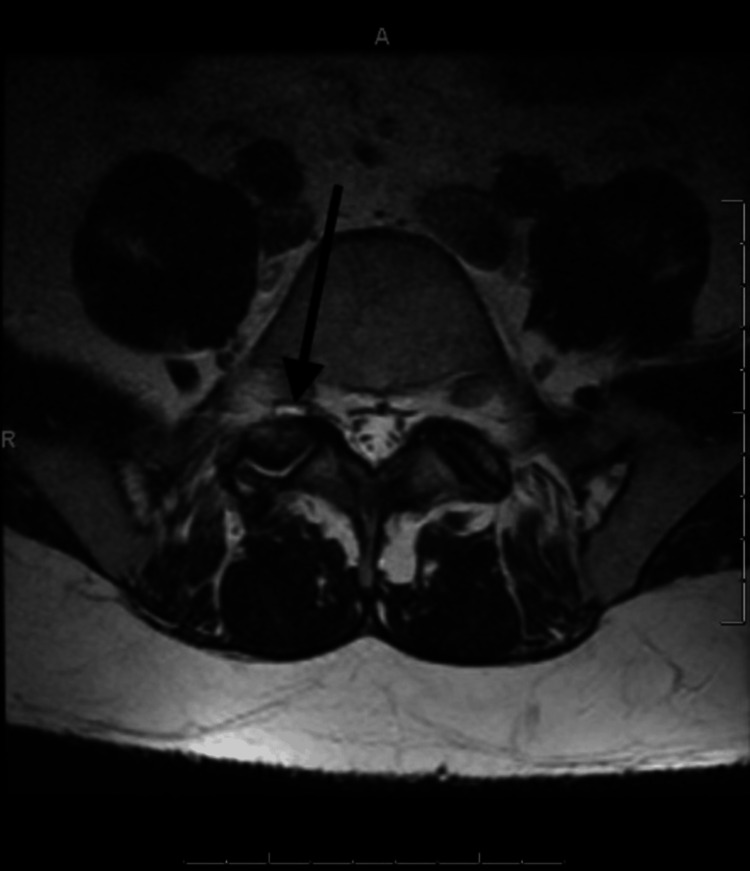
Axial MRI view of the lumbar spine for Case 2. Black arrow indicates a subtle synovial cyst at L5-S1. L5: Lumbar Segment 5; S1: Sacral Segment 1.
